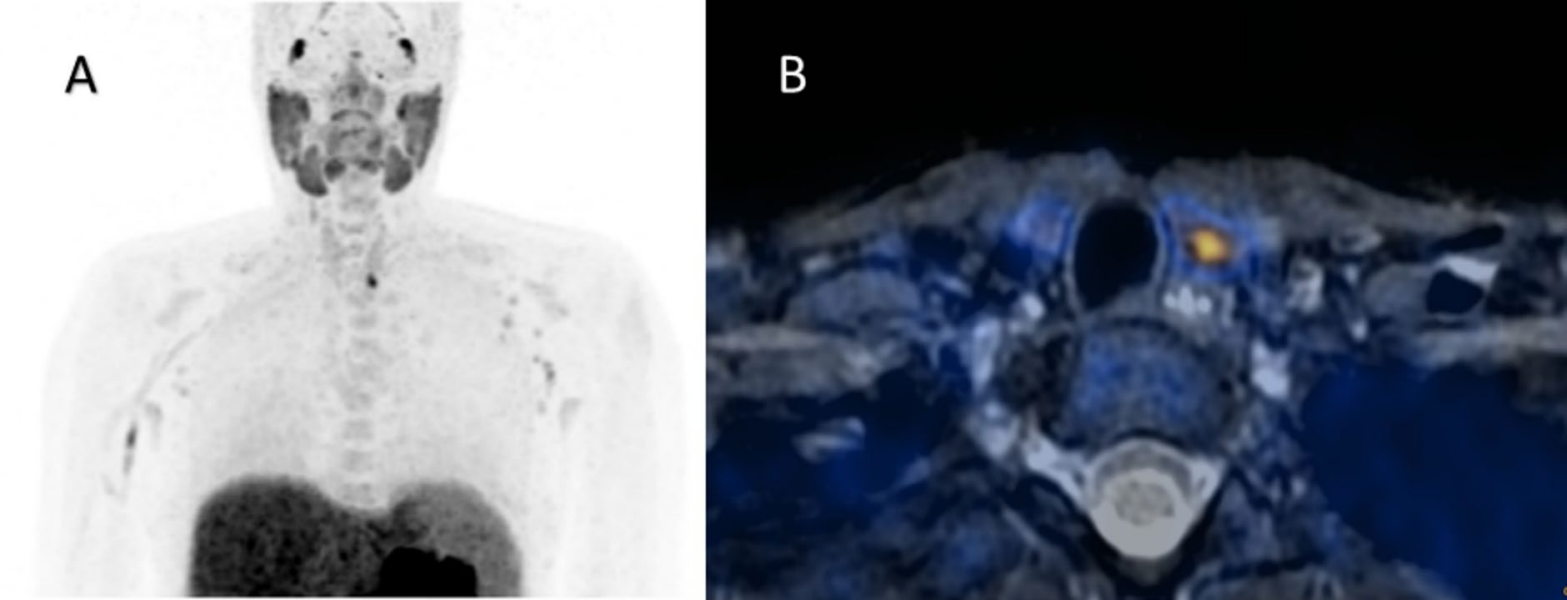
Intrathyroidal parathyroid adenoma. Legend:24-year-old woman with pHPT, albumin-corrected serum calcium level 2.64 mmol/L (2.19 – 2.54 mmol/L), PTH 107.1 ng/L (15-65 ng/L). MIP (A) and T2w-weighted fat-suppressed axial PET/MR (B) image show a left-sided intrathyroidal, intraparenchymal parathyroid adenoma (SUVmax 13.0, 6x4x6mm), confirmed by surgery
